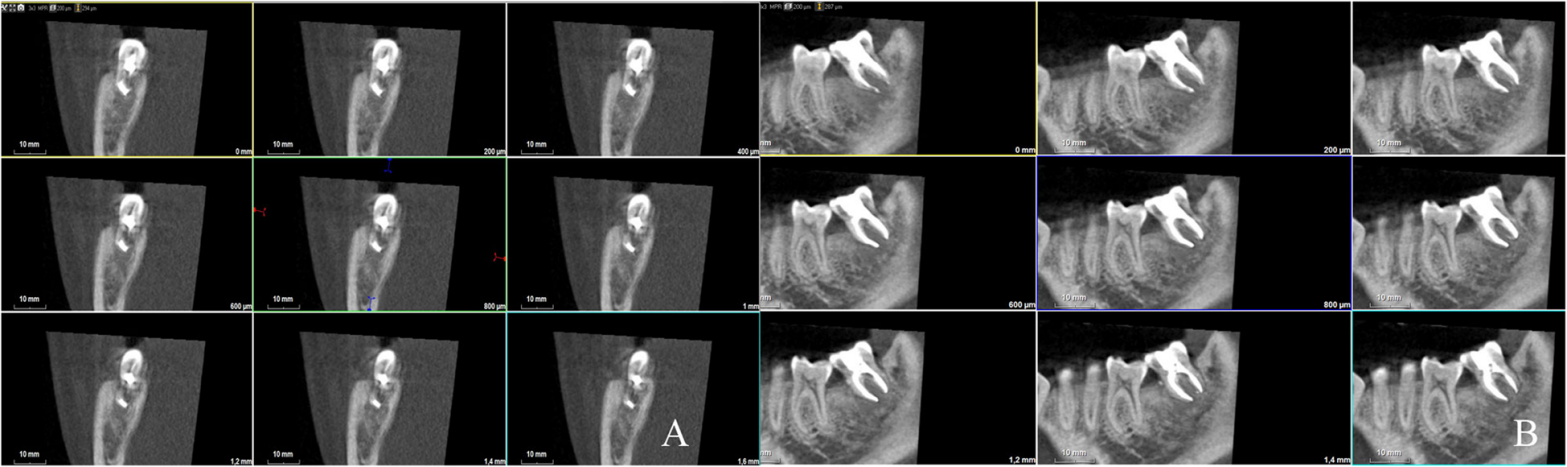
a Axial view in CBCT (White Fox) 24 months follow-up. b Sagital view in CBCT (White Fox) 24 months follow-up reveals bone regeneration
